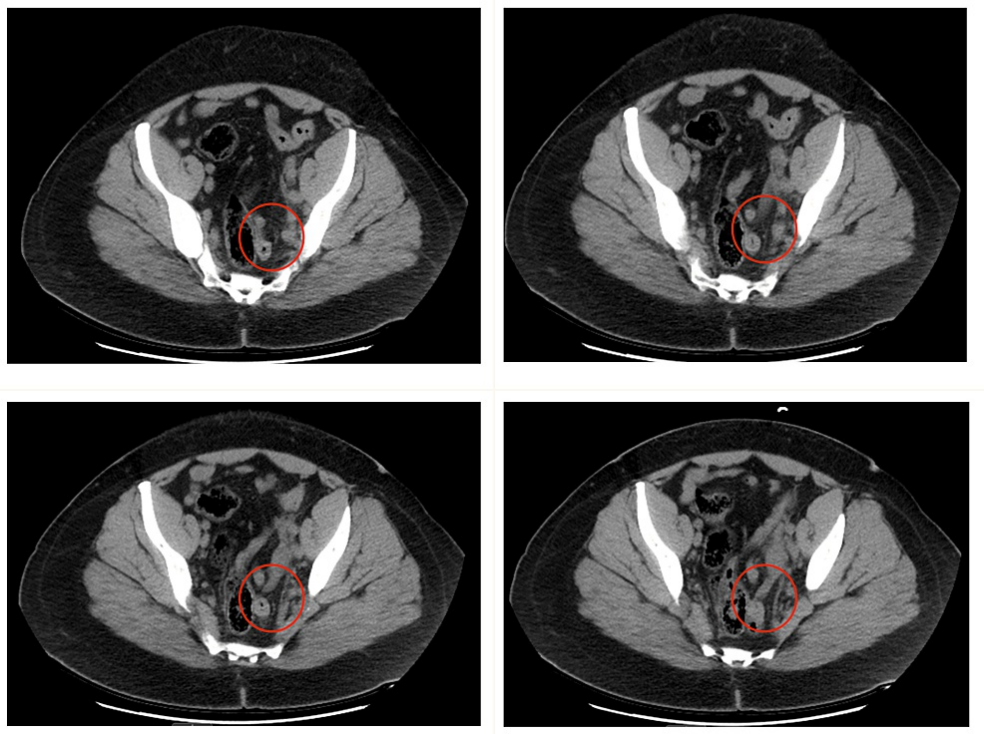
Transverse CT scan depicting appendicitis at different levels The appendix (depicted by the red circle) extends to and is inseparable from the left ovary. There is no adjacent inflammatory change CT: computed tomography
